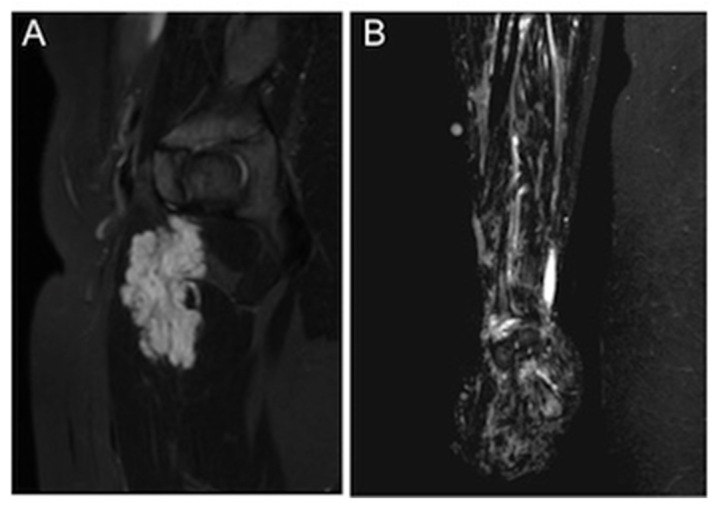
An example of the two different morphological appearances of VMs in MRI. (A) A discrete and well-demarcated VM in the thigh muscles. (B) A diffuse VM of the right forearm.
